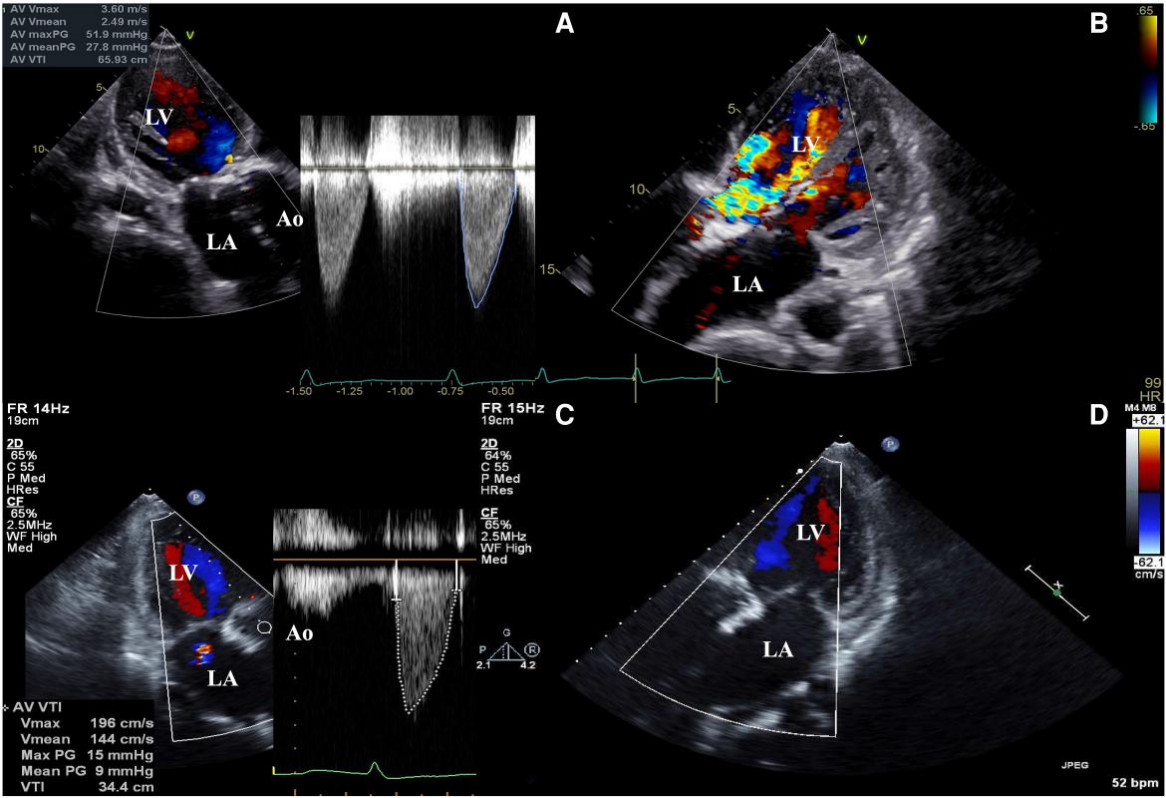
Periprocedural echocardiography. (*A*) Aortic valve assessment using continuous wave Doppler before TAVI. (*B*) AR grade before TAVI. (*C*) Aortic valve assessment using continuous wave Doppler after TAVI. (*D*) AR grade after TAVI. AR, aortic regurgitation; LA, left atrium; LV, left ventricle; TAVI, transcatheter aortic valve implantation.
